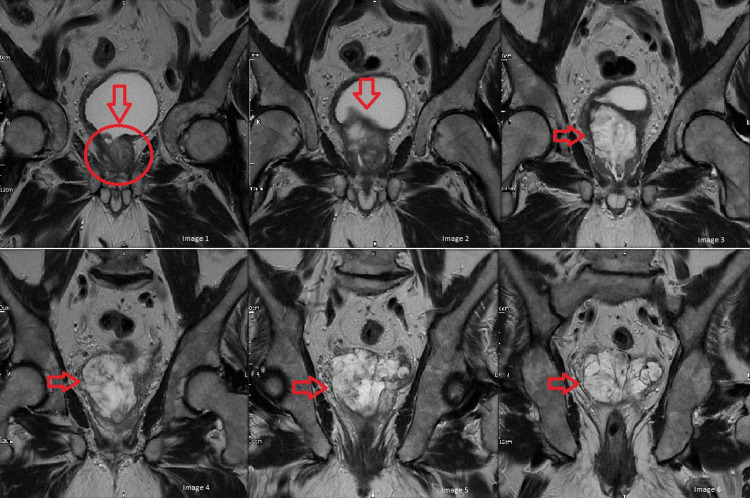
Images 1 to 6 (labeled) are part of a series of MRI images of the prostate showing heterogeneous, calcified mass inseparable from the ejaculatory ducts, distal vas deferens, and seminal vesicles bilaterally. The mass contains a large volume of hemorrhagic/proteinaceous material
